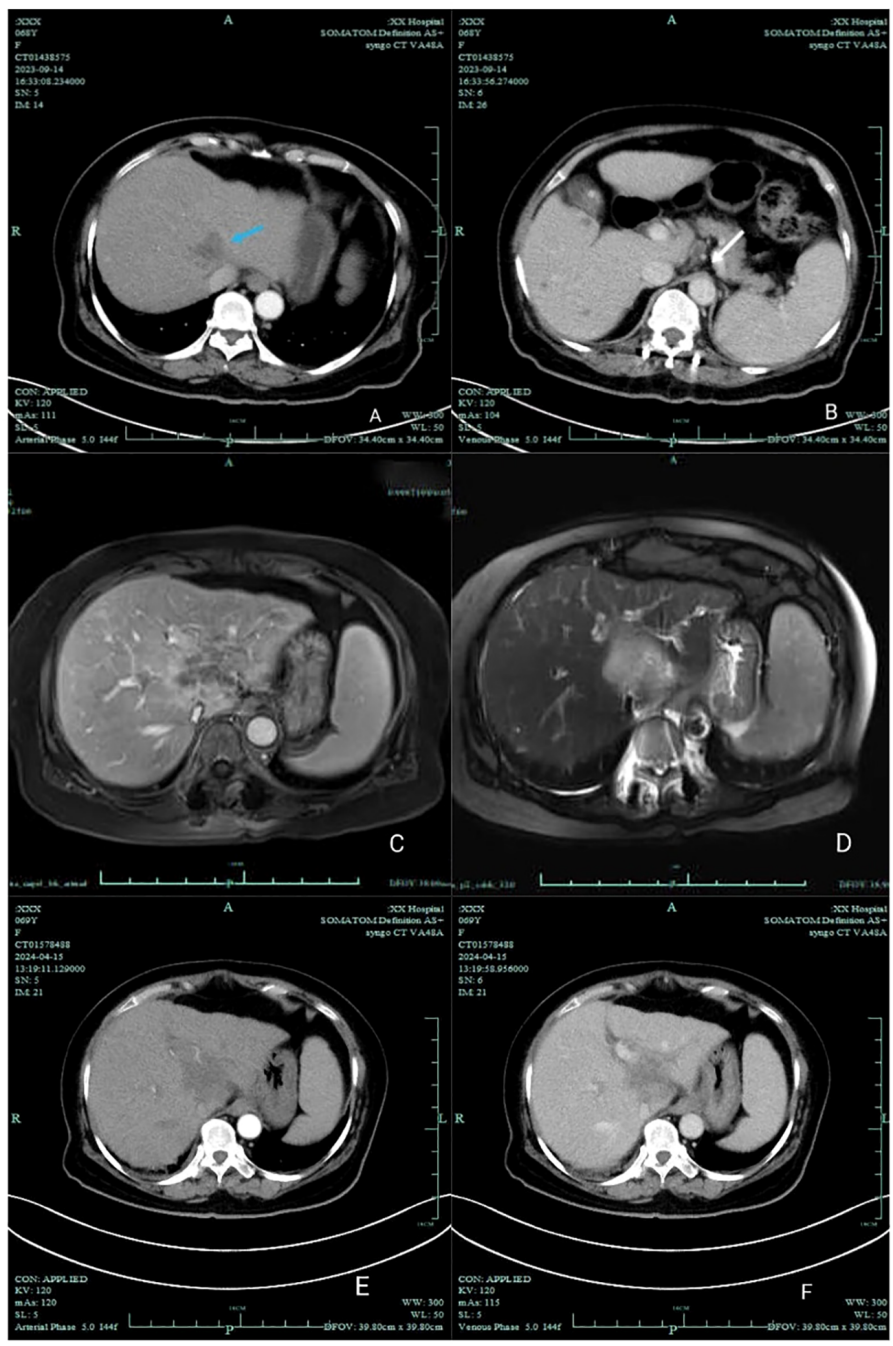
CT and MRI changes of patients. In September 2023 (A, B), (A) showed a marked reduction in tumor vascularity. (B) showed multiple mildly enlarged lymph nodes around the celiac trunk. The largest lymph node measured approximately 1.0 cm in short-axis diameter, representing a 57% reduction compared to Figure 1. In March 2024 (C, D), the tumor showed progression following targeted therapy and immunotherapy. It measured approximately 4.7 × 3.9 cm, representing an 88% increase from previous imaging. By April 2024 (E, F), further tumor progression was observed, accompanied by lymph node enlargement.
